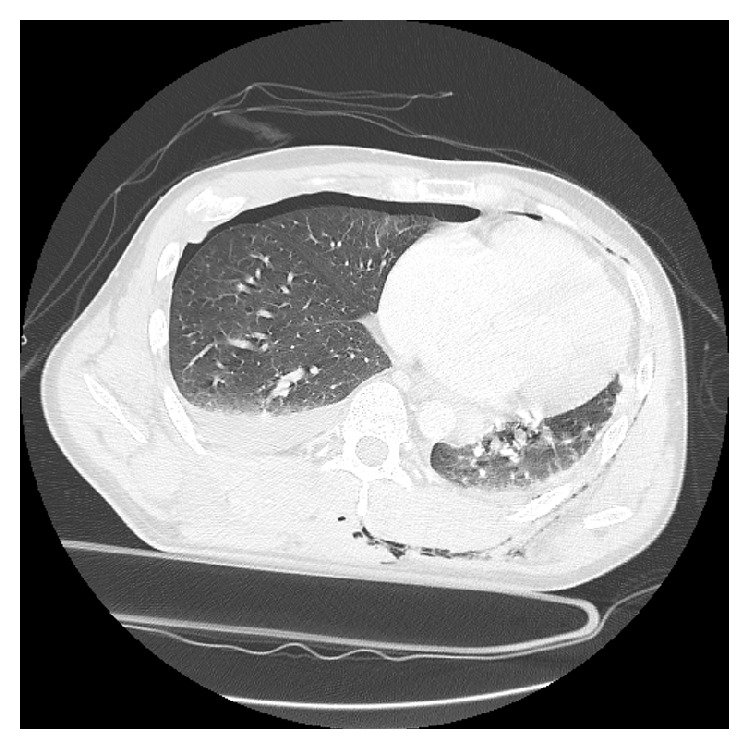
Preoperative CT image of the chest. Multiple rib fractures and a right hemopneumothorax.
